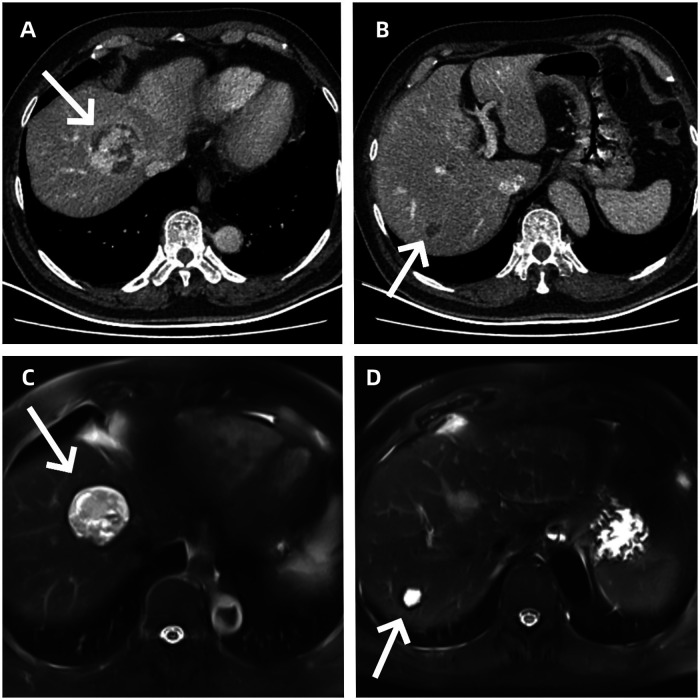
(A) enhanced CT shows a tumor in the left medial lobe of the liver. (B) Enhanced CT shows a tumor in the lower segment of the right posterior lobe of the liver. (C) MRI shows a tumor in the left medial lobe of the liver. (D) MRI shows a tumor in the lower segment of the right posterior lobe of the liver.
